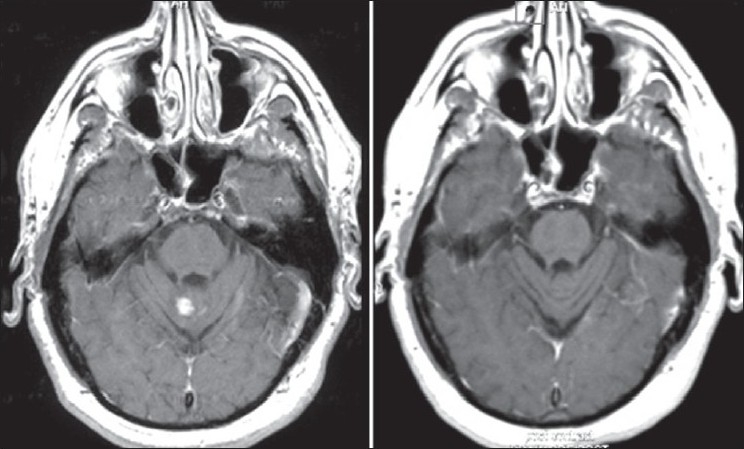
Axial T1-weighted postcontrast images demonstrating enhancing lesion in the vermis 8/06 (left) with resolution of the lesion post infl iximab 3/07 (right)
